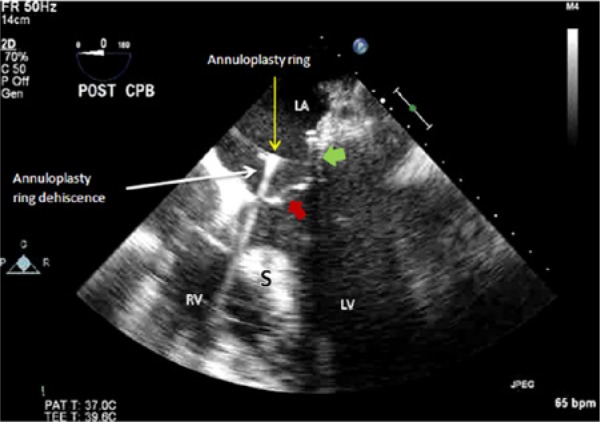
Two-dimensional transesophageal echocardiography midesophageal 5-chamber view showing dehiscence of the annuloplasty ring, with the ring causing echo dropout. The red arrow shows the anterior mitral valve leaflet, and the green arrow shows the posterior mitral valve leaflet. Abbreviations: S, interventricular septum; RV, right ventricle; LV, left ventricle.
